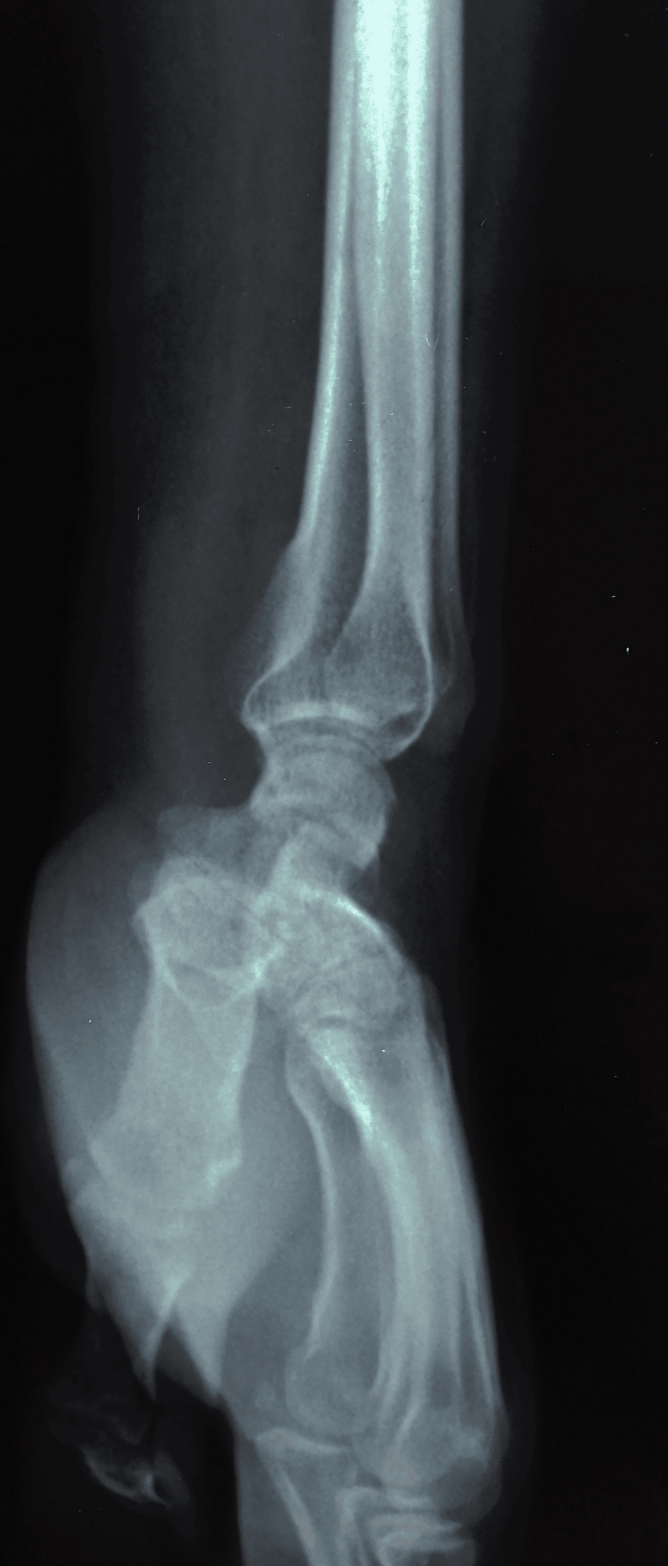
Radiograph showing lateral view of the right wrist and hand
